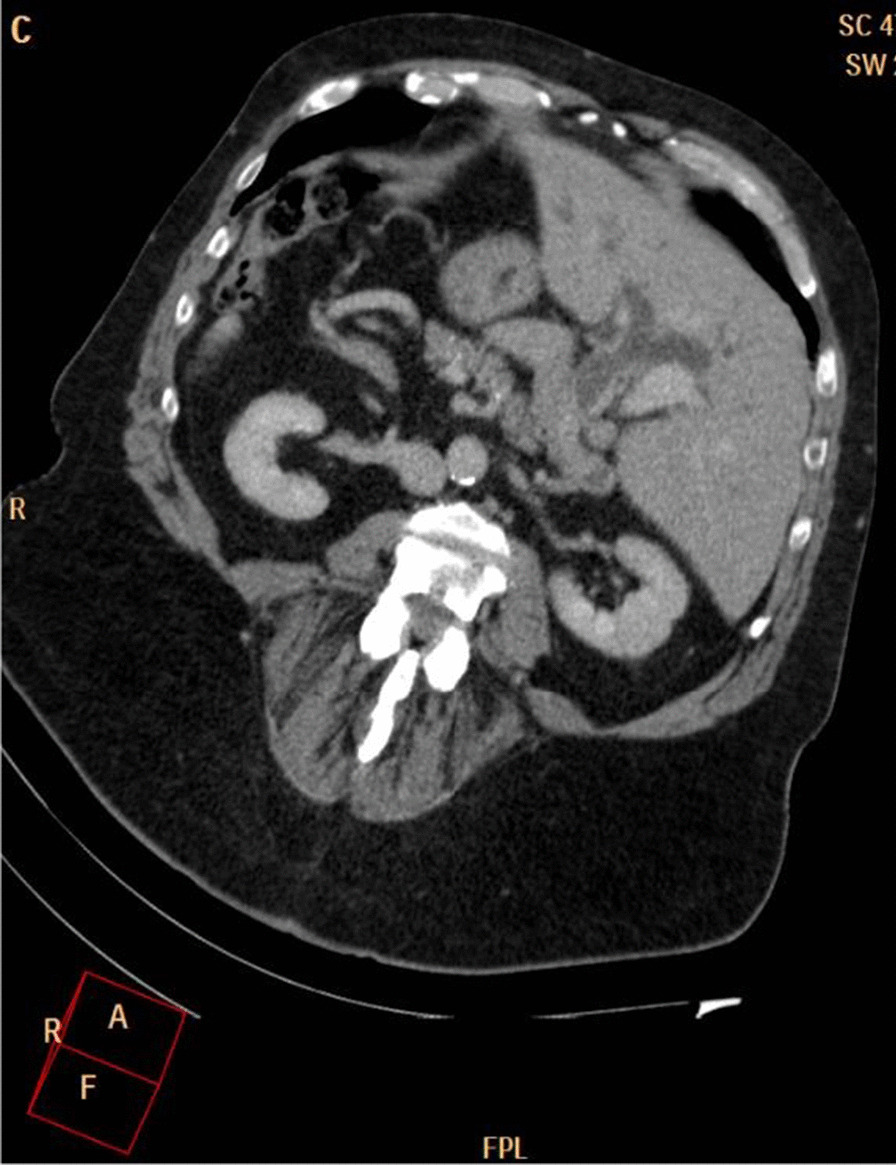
Preoperative CT scan: the liver is located on the left side, the intra- and extrahepatic bile ducts are dilated
